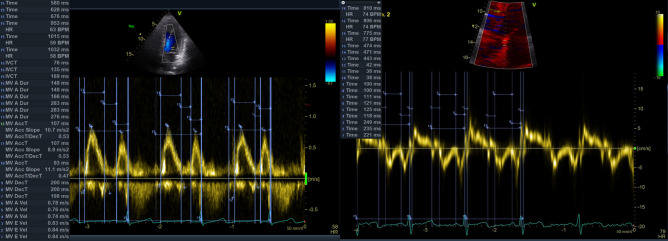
Exemplary echocardiography tracings at a PEEP level of 10 cm H_2_O. The frames that correspond to the PV-Loop family in Fig. 1 are shown. The left panel shows a transmitral pulsed wave Doppler trace, the right panel a tissue Doppler tracing
